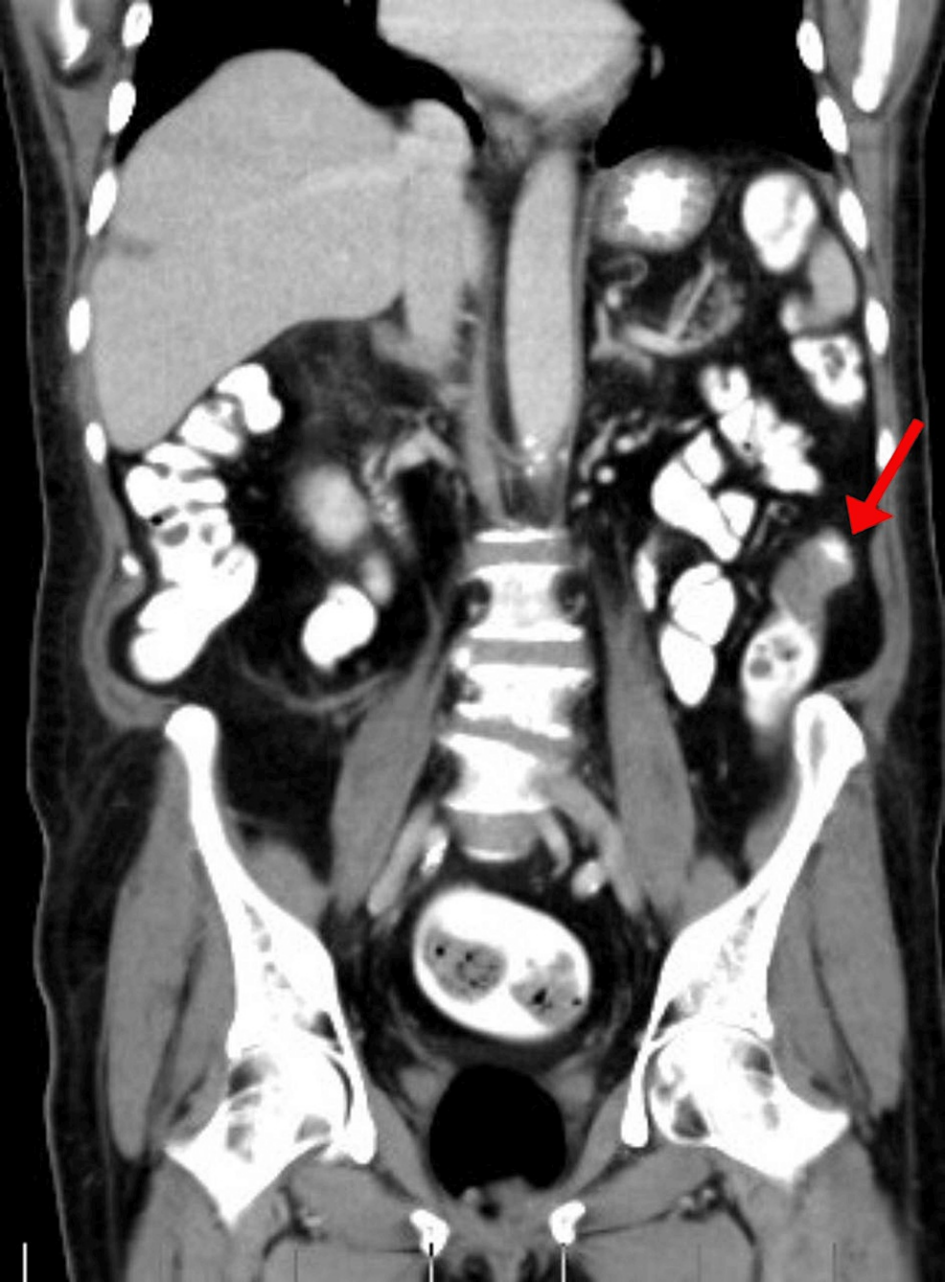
Coronal view CT Abdomen with IV contrast. Mural circumferential thickening at the at inferior portion of the descending colon near the distal sigmoid 3.5 cm in length.
